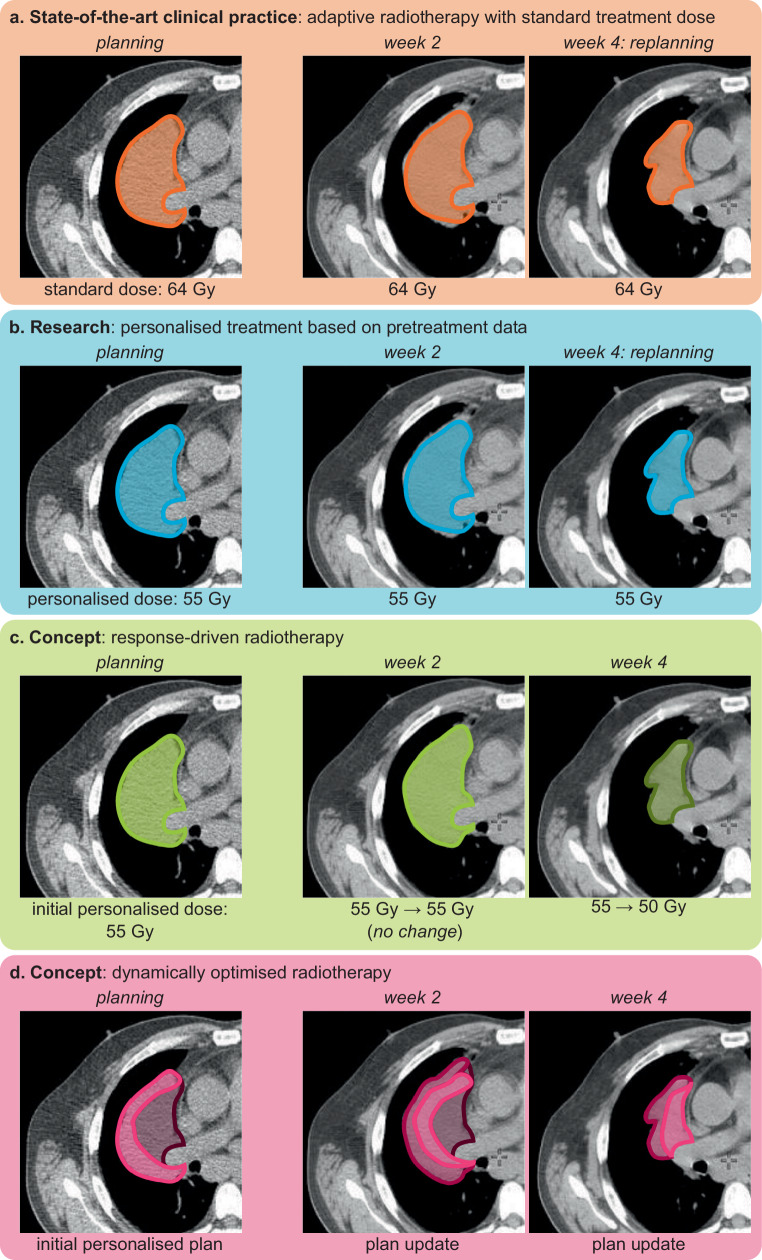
Concepts for personalising radiotherapy using artificial intelligence. The current state of the art does not involve artificial intelligence (AI) systems for personalisation (a). A standard treatment dose is prescribed and delivered in an adaptive fashion with replanning. Current research focuses on AI systems that personalise radiotherapy based on pretreatment data (b). In this example, the AI system predicts that the prescribed dose may be reduced, while still achieving an effective treatment. The treatment is then delivered in a conventional manner. In response-driven radiotherapy, the initial plan uses predictions of the AI system based on pretreatment data (c). As data are acquired during treatment, the AI system uses the observed tumour response to alter the treatment. In this case the good tumour response prompts a reduction in the total dose. Dynamically optimised radiotherapy uses an AI system to dynamically optimise treatment and achieve a curative effect while minimising the dose delivered to normal tissues (d). In the presented example, the AI system initially prescribes a higher dose to the outer tumour rim and gradually shifts the high-dose region towards the heart. Both response-driven and dynamically optimised radiotherapy are conceptual, and relatively little research has been done. CT images from Chen et al. [5] under CC BY 4.0 licence
